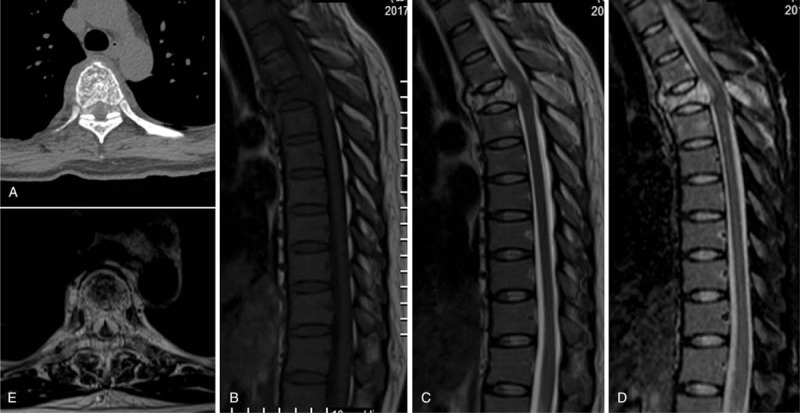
(A) Computed tomography shows sclerosis of the cancellous bone and a fracture involving the anterior cortex of the vertebral body.(B) Magnetic resonance imaging (MRI) showsa compression fracture of the T5 vertebra.(C) MRI shows T5 vertebral body compression in the dural sac and spinal cord.(D) MRI showsahemangioma of the T5 vertebral body and hyperintensity of the spinous process. (E) MRI shows an extraosseous component of the hemangioma (arrow), indicating an aggressive vertebral hemangioma.
